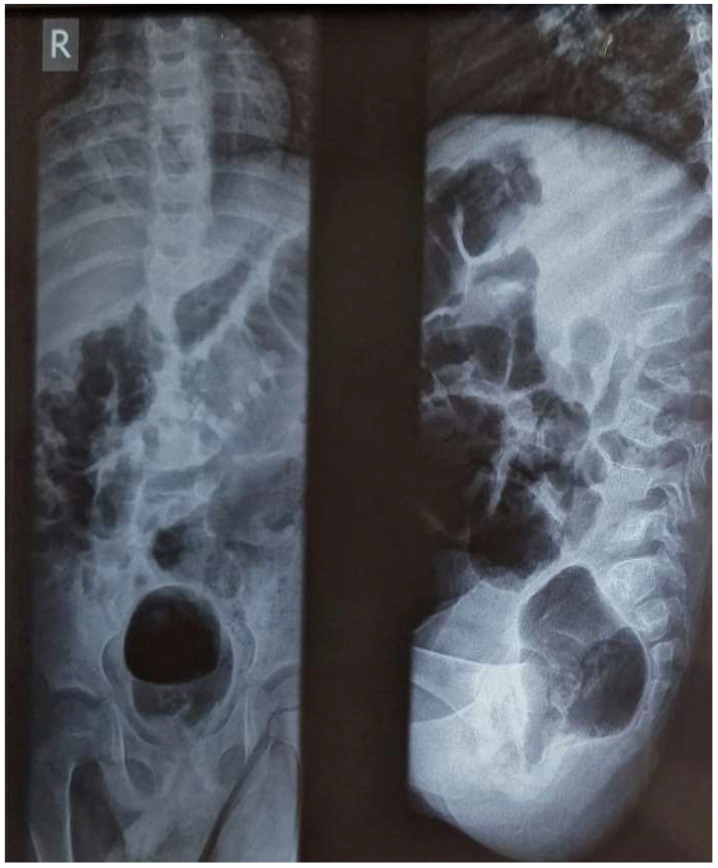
Plain X-ray of the spine.
